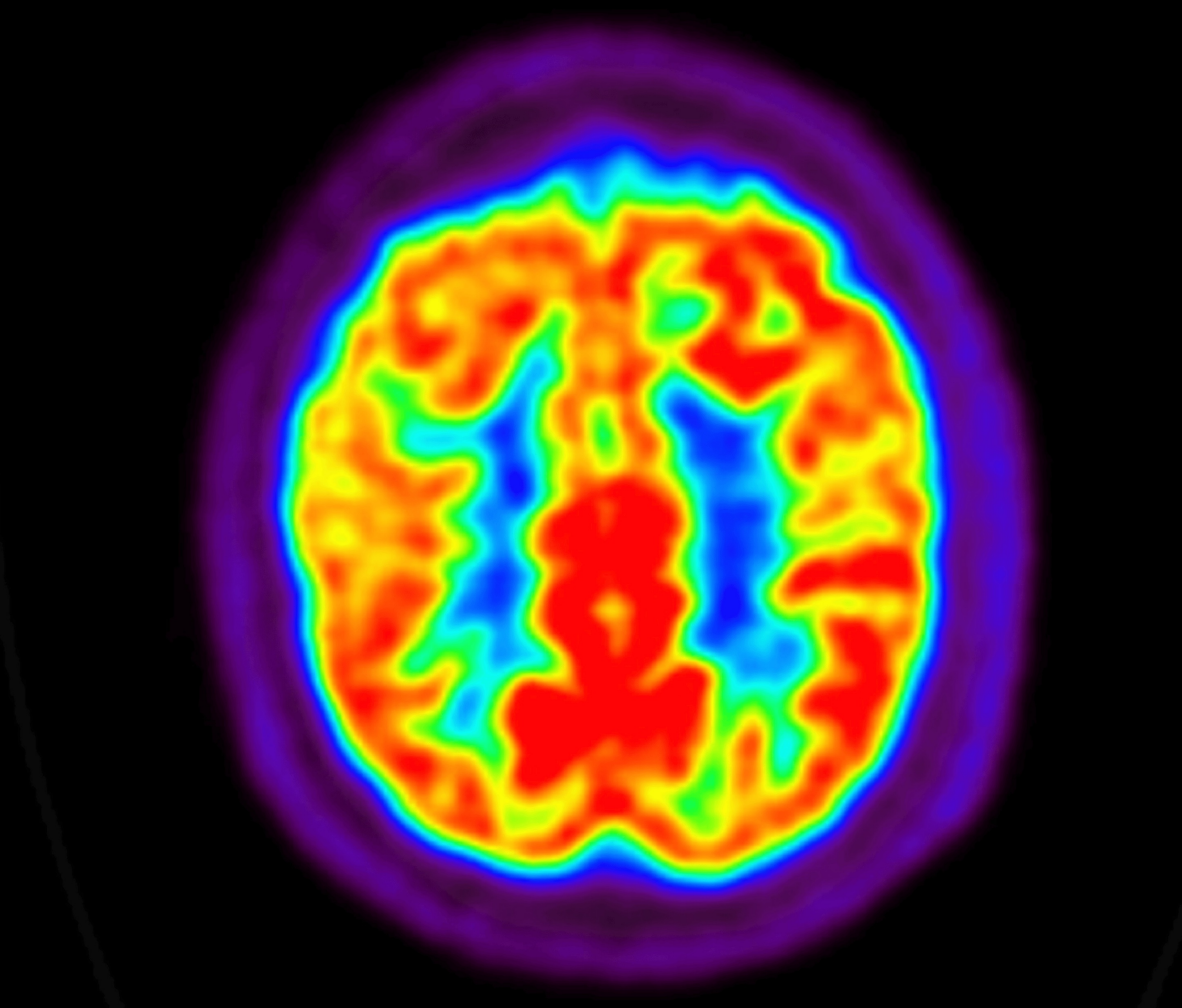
Fluorodeoxyglucose-positron emission tomography (FDG-PET) scan Normal metabolism of glucose.
